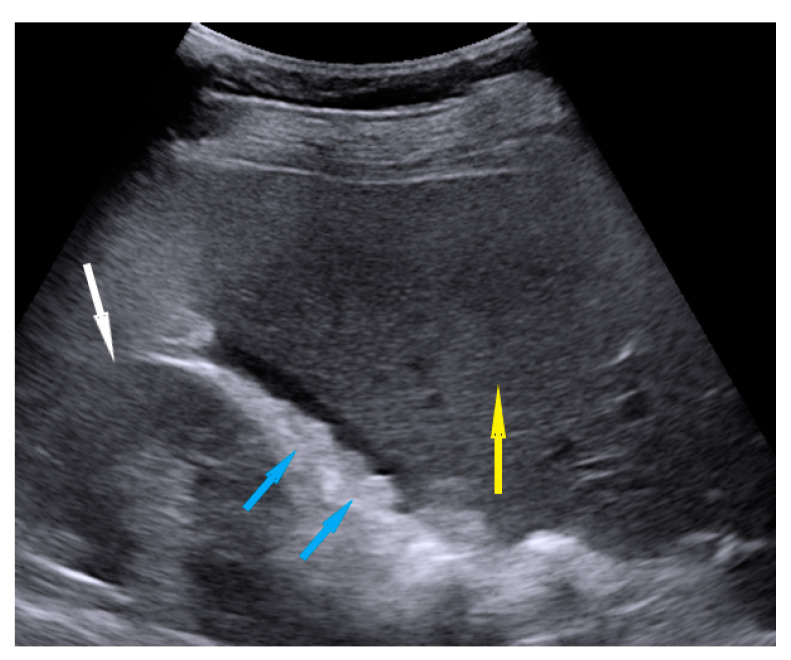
Ultrasound image of nodules on the peritoneum of Morison pouch. Blue arrows identify nodules, yellow—liver, white—kidney.
